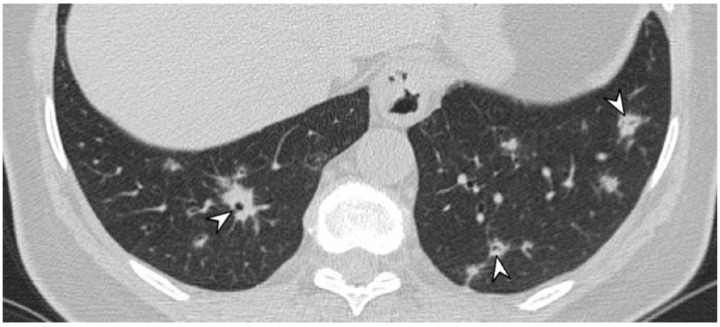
A patient with diagnosis of Wegener’s disease. CT image shows multiple bilateral lung nodules (arrowheads), with air bronchogram sign.
